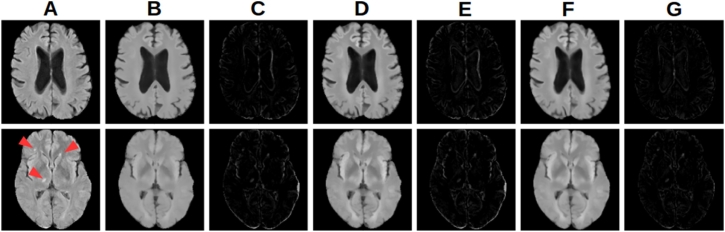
Effects of intensity correction and registration of synthetic images on a (top) pathology free and (bottom) pathological subject. (A) FLAIR image. (B) Rigidly registered synthetic image. (C) Difference image from (A) to (B). (D) Rigidly registered intensity corrected synthetic image. (E) Difference image from (A) to (D). (F) FFD registered intensity corrected synthetic image. (G) Difference image from (A) to (F). Note that the intensity correction and FFD registration do not prevent detection of the pathology (arrows).
